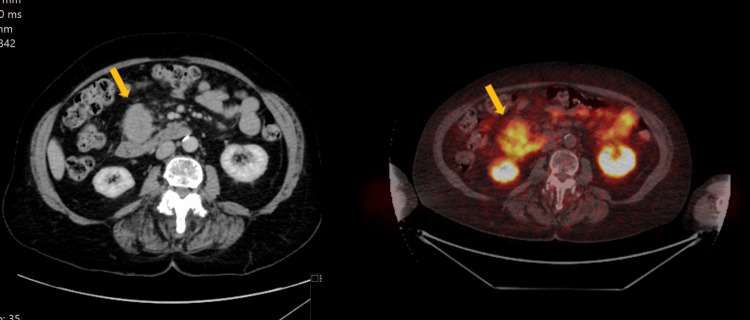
Abdominal mass on work-up, proven to represent UCD. On the left, a CT scan demonstrates a mass (yellow arrow) lying adjacent to the pancreatic head, causing a pressure effect on the adjacent renal vasculature. On the right, an FDG PET scan shows avidity of the mass (yellow arrow). UCD: Unicentric Castleman disease; FDG PET: Fluorodeoxyglucose positron emission tomography.
